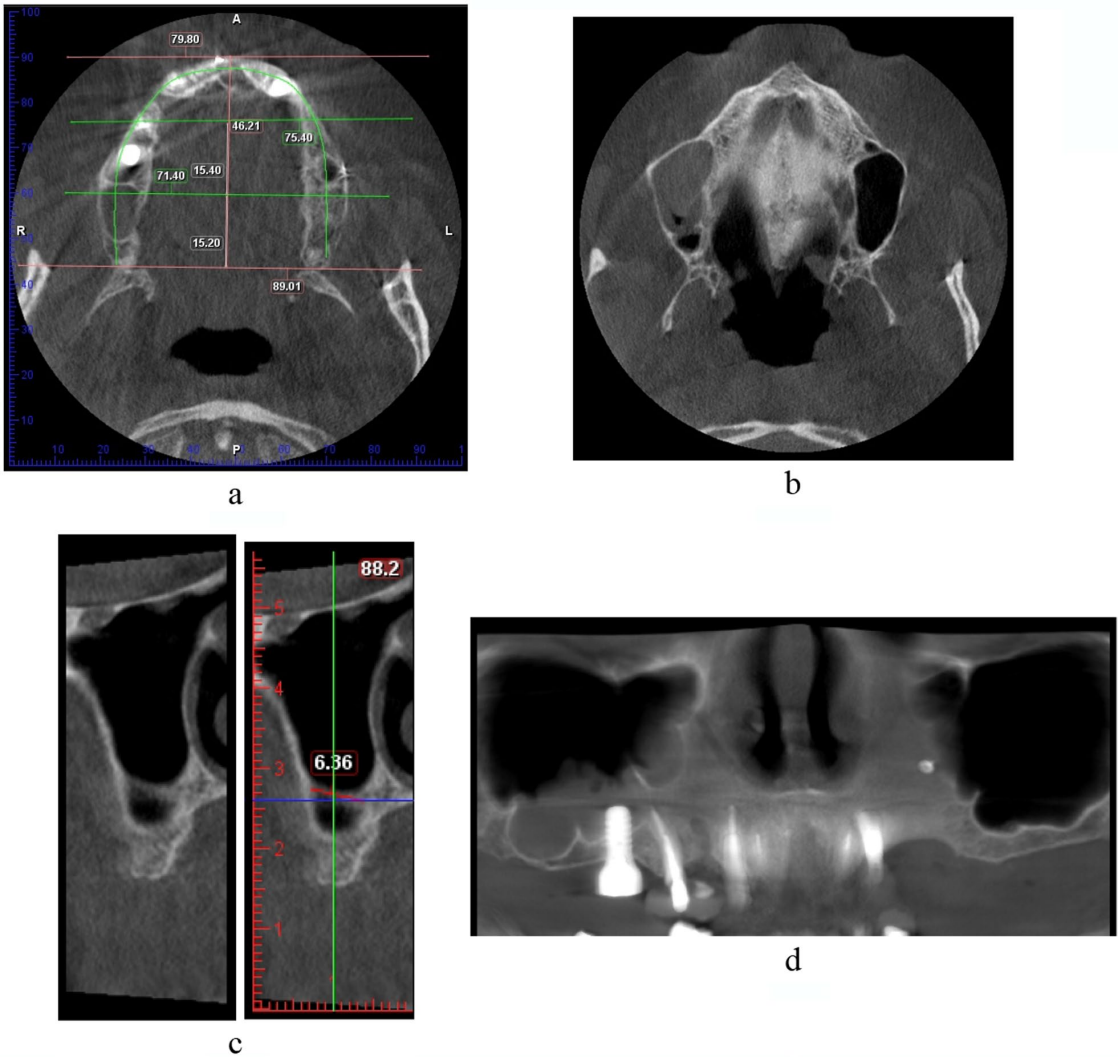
CBCT sections of a dentate patient. a Axial section where the maxillary jaw is divided into three sections. b Detection of a septum in the middle third of the sinus. c Cross-sectional cuts showing the orientation and length of the detected septum (with and without measurement). d Panoramic view showing multiple septa in the lower compartment. *Note* the detection of the other two septa in the medial wall of the sinus.
